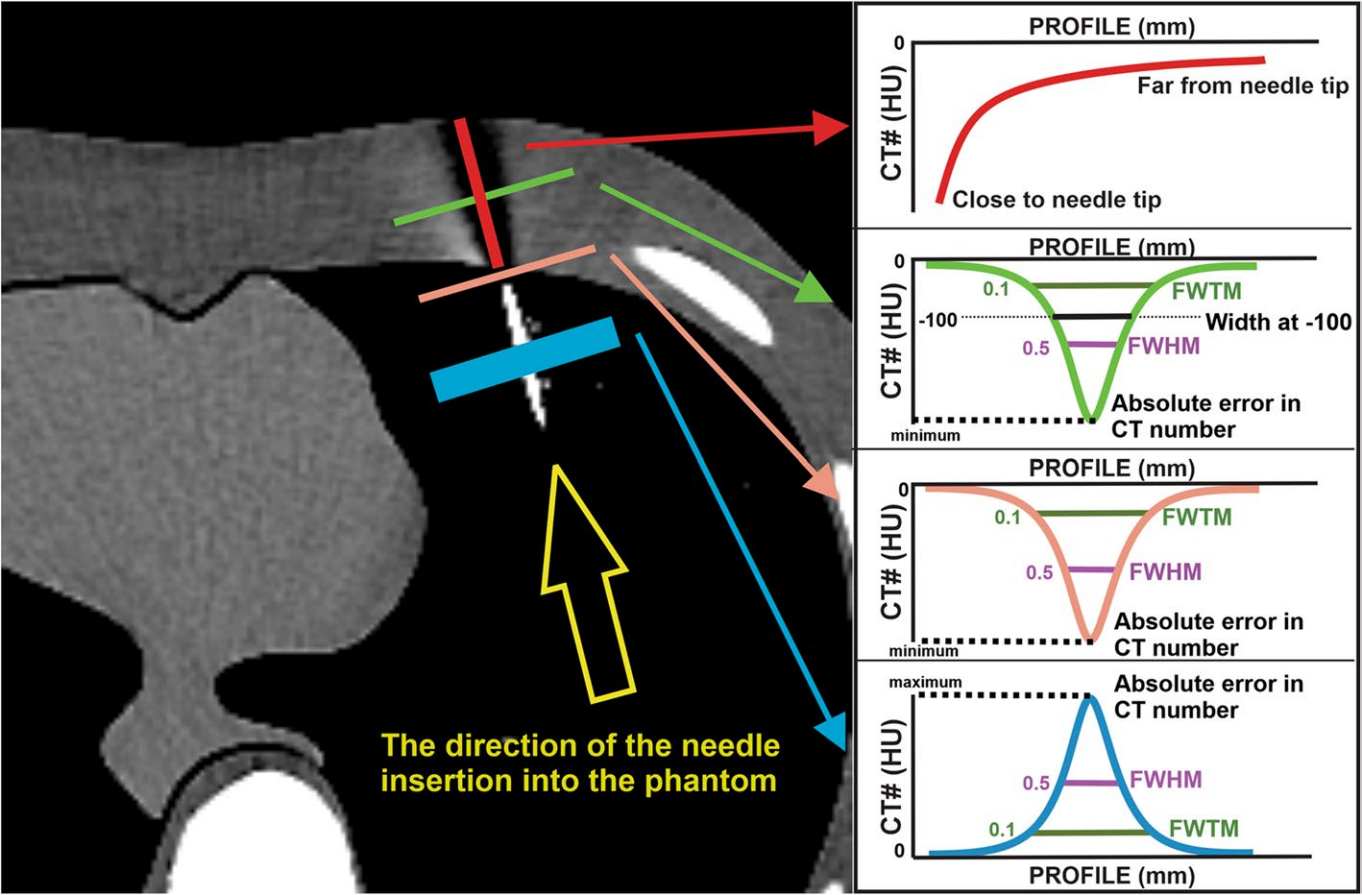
Shown are the positions in the CT image where the profiles were drawn to obtain the values of FWHM, FWTM, and the absolute error in HU used to quantify the artifacts generated from the biopsy needle. FWHM = full width half maximum, and FWTM = full width at one-tenth of maximum (FWTM). The needle was inserted from the back of the right lung until the needle tip reached the region of tissue at chest level
